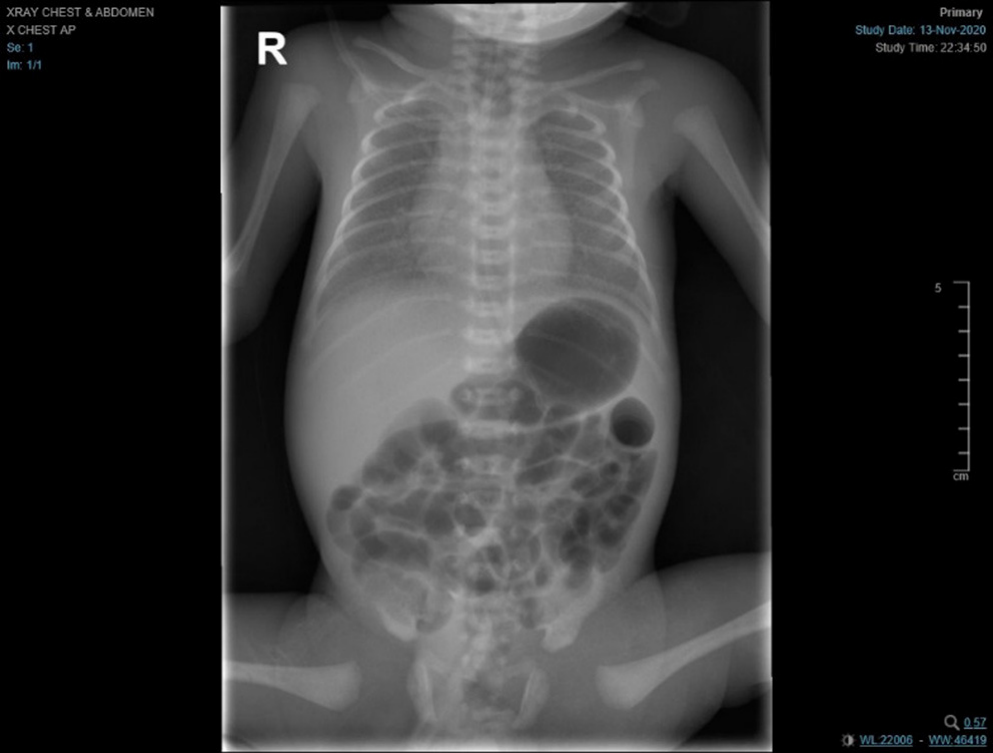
Chest radiograph showed bilateral ground glass appearance with bilateral haziness and good lung volume.
